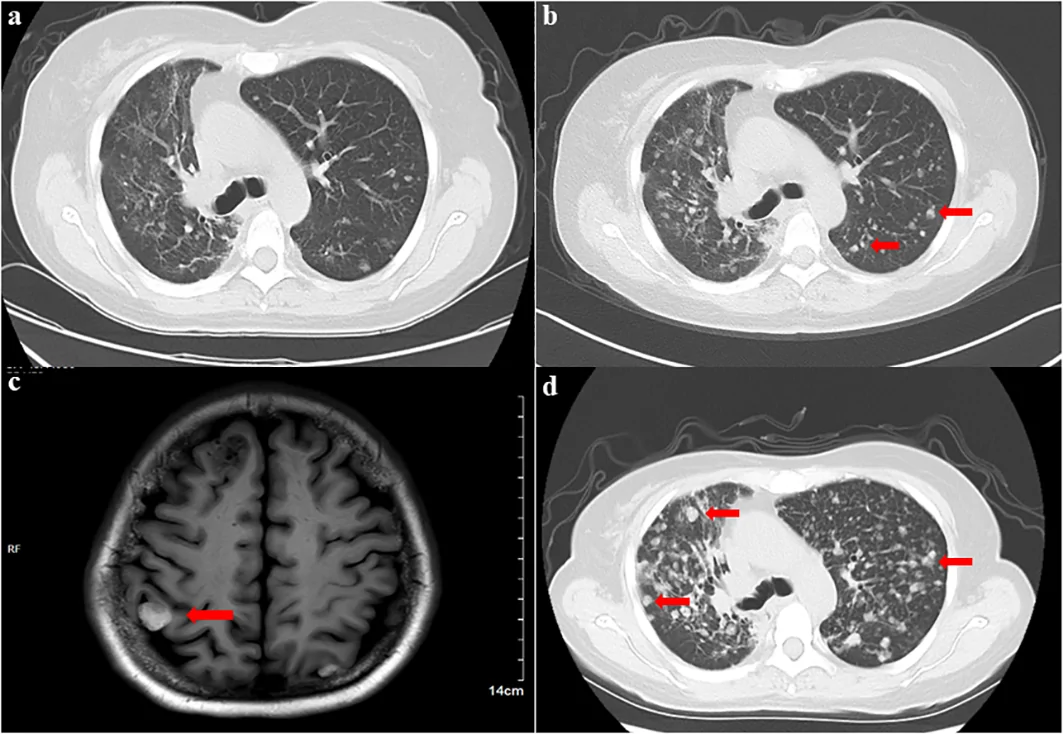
Chest CT scan showing (a) a reduction in the number and size of metastases in bilateral lungs with the 7^th^-line treatment with pertuzumab, trastuzumab, and albumin-bound paclitaxel. New metastatic lesions (b) in bilateral lungs can be seen in subsequent chest CT scans, and (c) cerebral MRI showing a new enhancement lesion (arrow). Chest CT scan at the final follow-up shows (d) an increase in the number and size of metastases in bilateral lungs (arrows).
